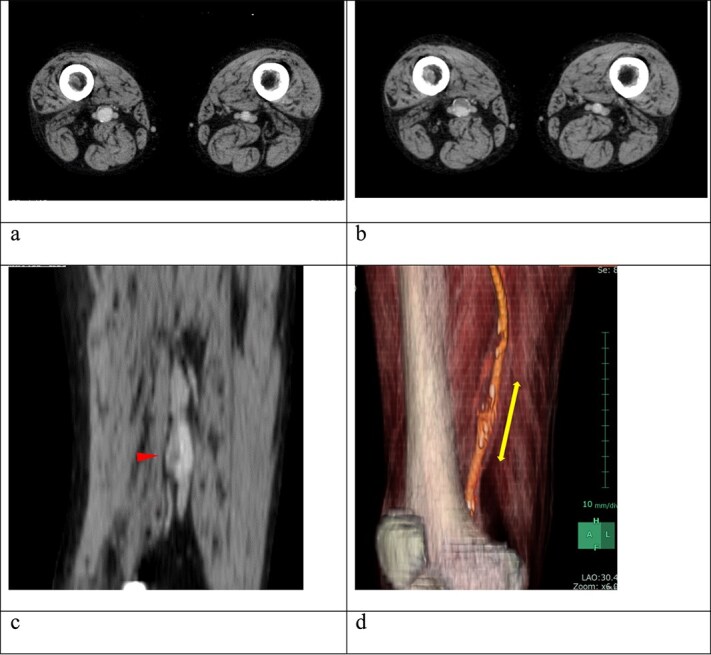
(a) Contrast-enhanced computed tomography (CT) performed 2 years earlier showed no thrombus in the right superficial femoral artery (SFA) aneurysm. (b) Despite ongoing oral anticoagulation therapy, preoperative contrast-enhanced CT revealed thrombus progression within the aneurysm, which was located 6 cm proximal to the knee and measured 15 mm in diameter. (c) The sagittal section of the contrast-enhanced CT showed an irregular thrombus on the inner wall of the aneurysm (arrow). (d) A three-dimensional-reconstructed contrast-enhanced CT identified the SFA aneurysm (arrow).
